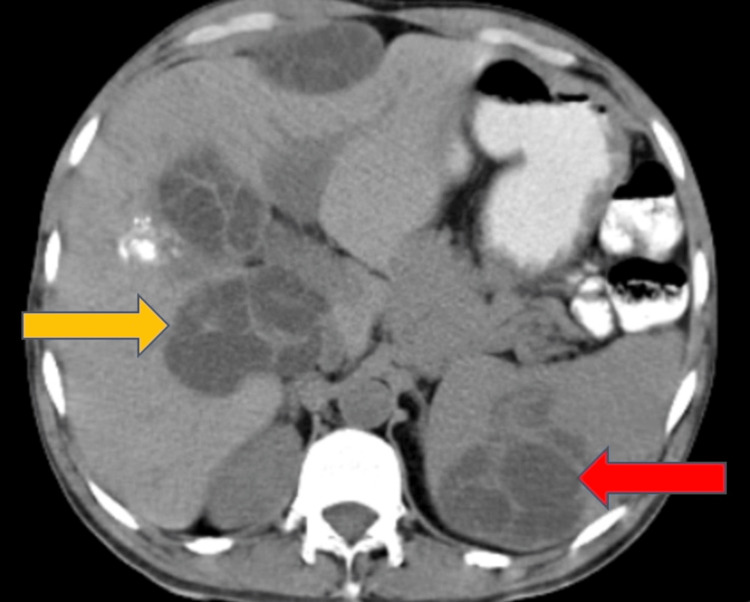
A CT of the abdomen showing hydatid cyst in the liver (yellow arrow) and the spleen (red arrow)
